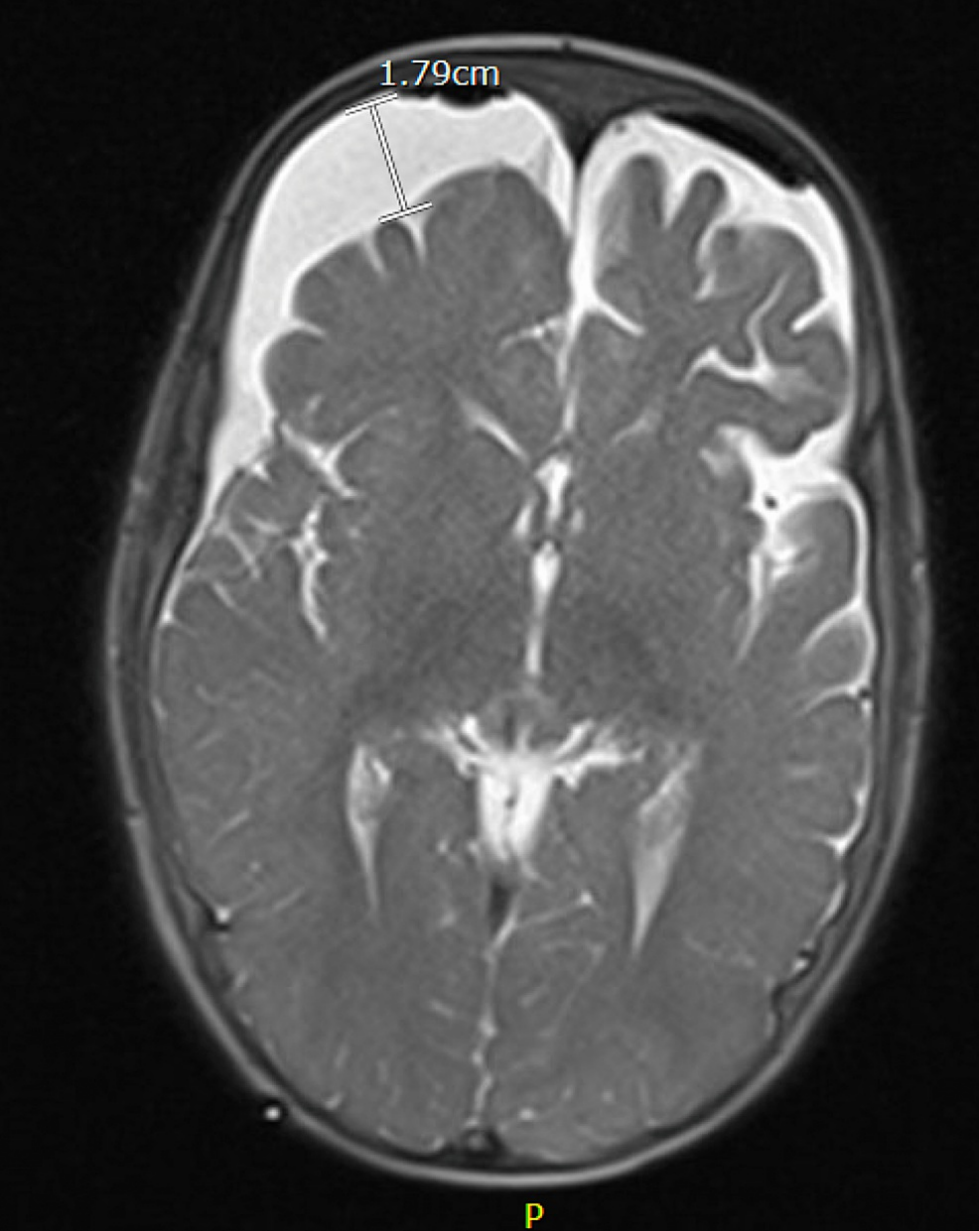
MRI of the brain T2 axial view showing persistent subdural collection, right greater than left, status post-clamping trial.
